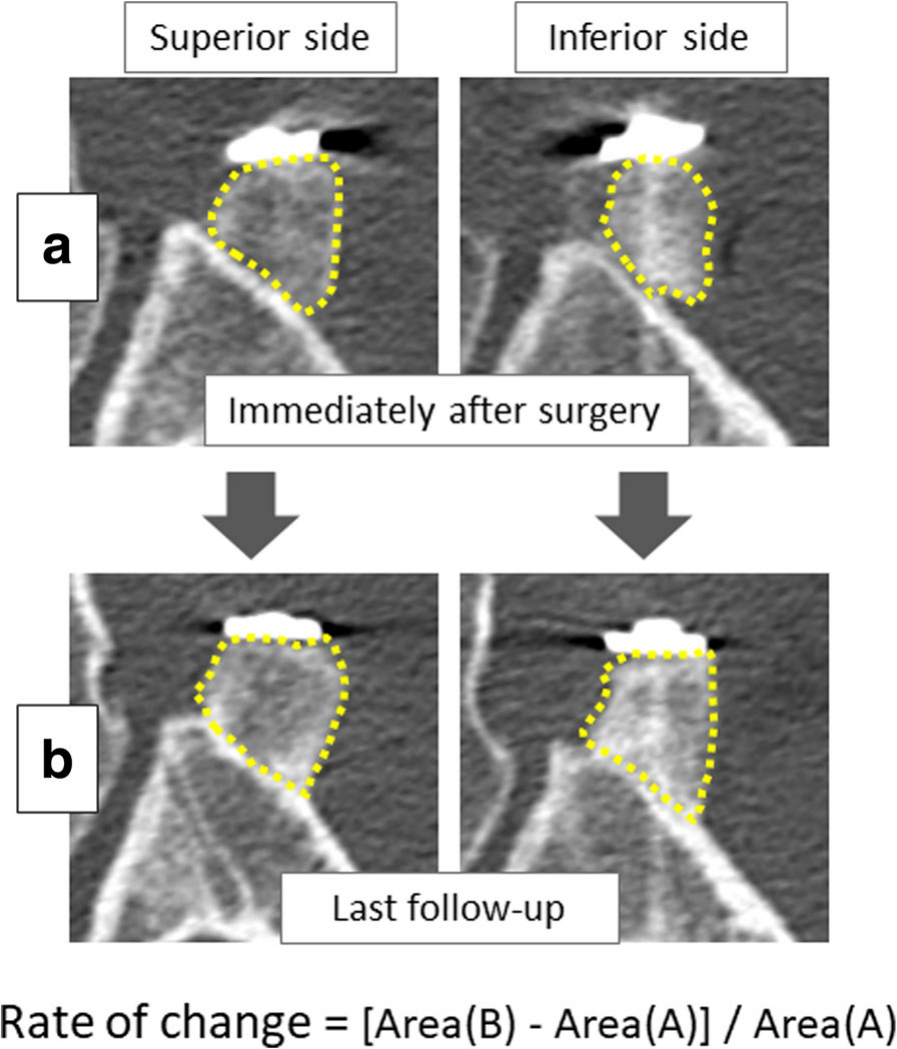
Measurement of changes in the surface area of the graft. Computed tomography scans were taken immediately postoperatively (a) and at the last follow-up (b). In the most superior and most inferior slices, respectively, the shape of the superior and inferior sides of the coracoid process graft was traced to measure their area. The rate of change in bone area was calculated for each side. The overall change in bone area was also calculated (i.e., averaged for the superior and inferior sides)
